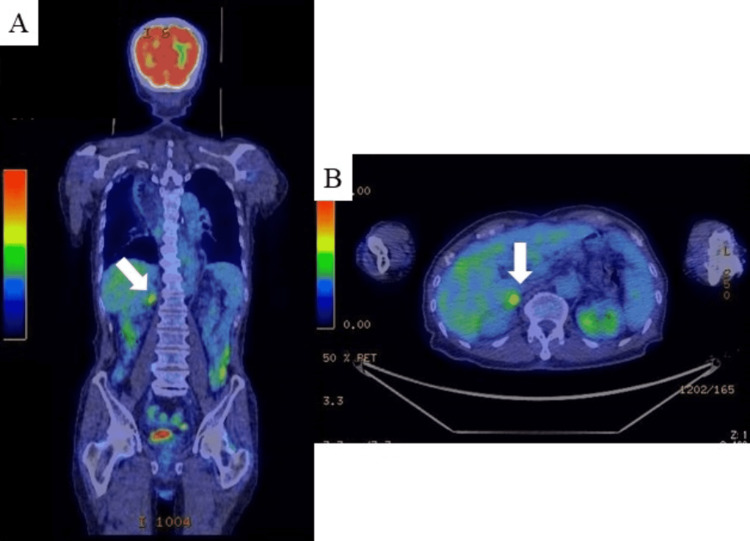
FDG positron emission tomography computed tomography after one-year surveillance period. (A and B) The images show remarkable FDG uptake in the right adrenal gland with an enlargement area of 20 mm (white arrows). FDG: fluorodeoxyglucose
